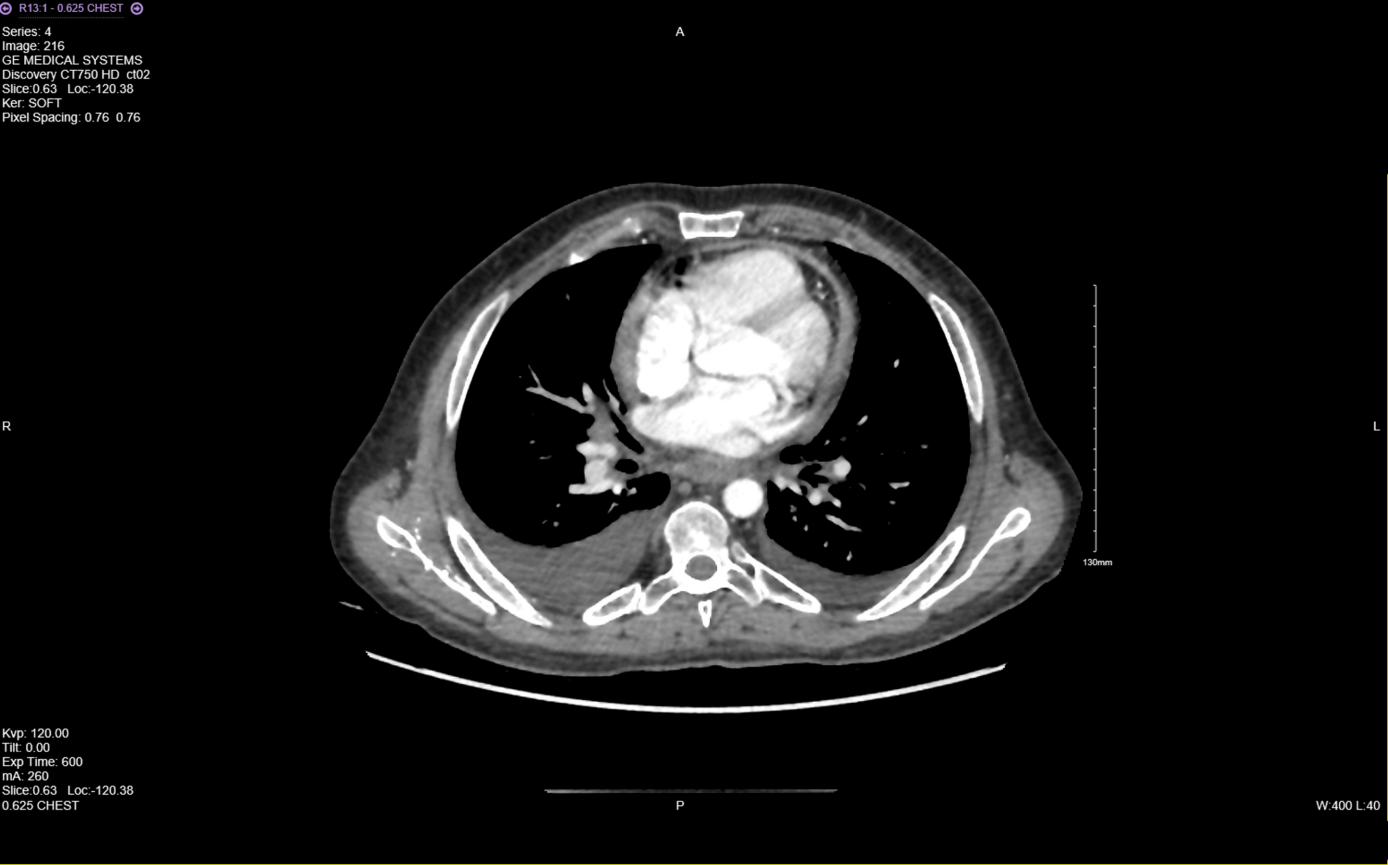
A CT scan conducted on 27/07/2023 showed bilateral mild to moderate pericardial effusion, along with significant pericardial thickening and enhancement.
